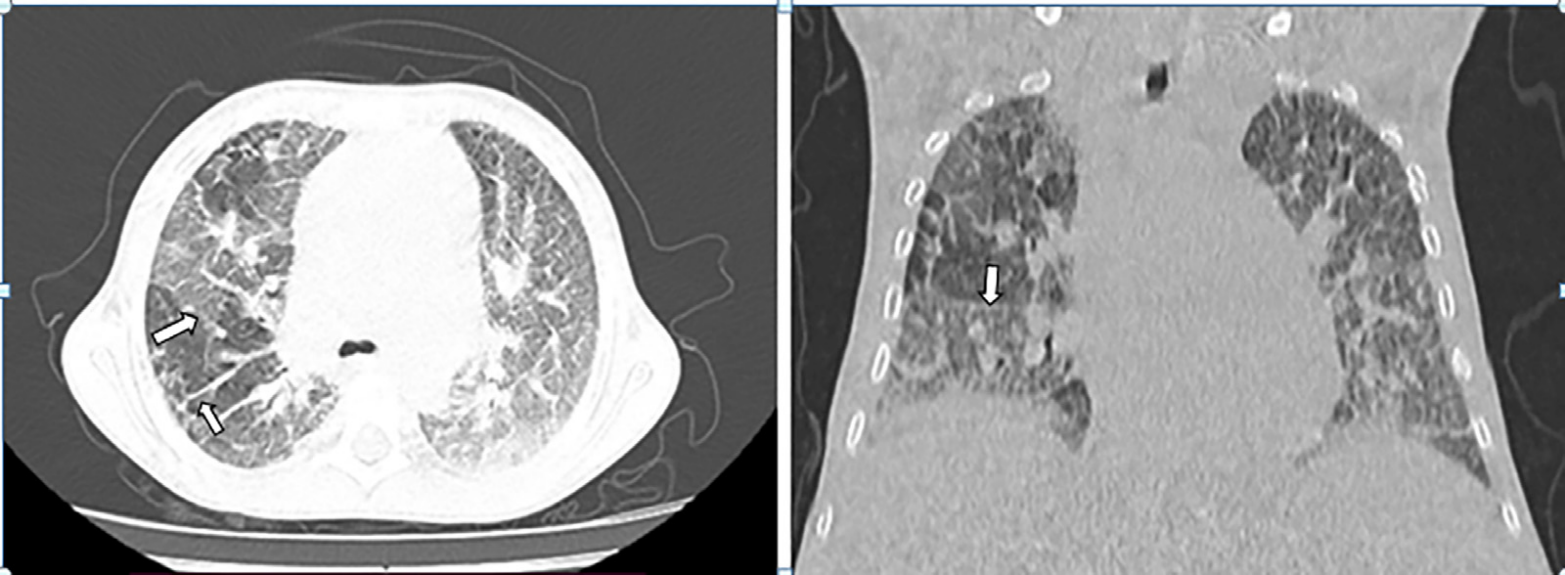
Interstitial lung disease. Axial and coronal chest CT scan showing diffuse septal thickening and ground glass opacities (Arrows).
